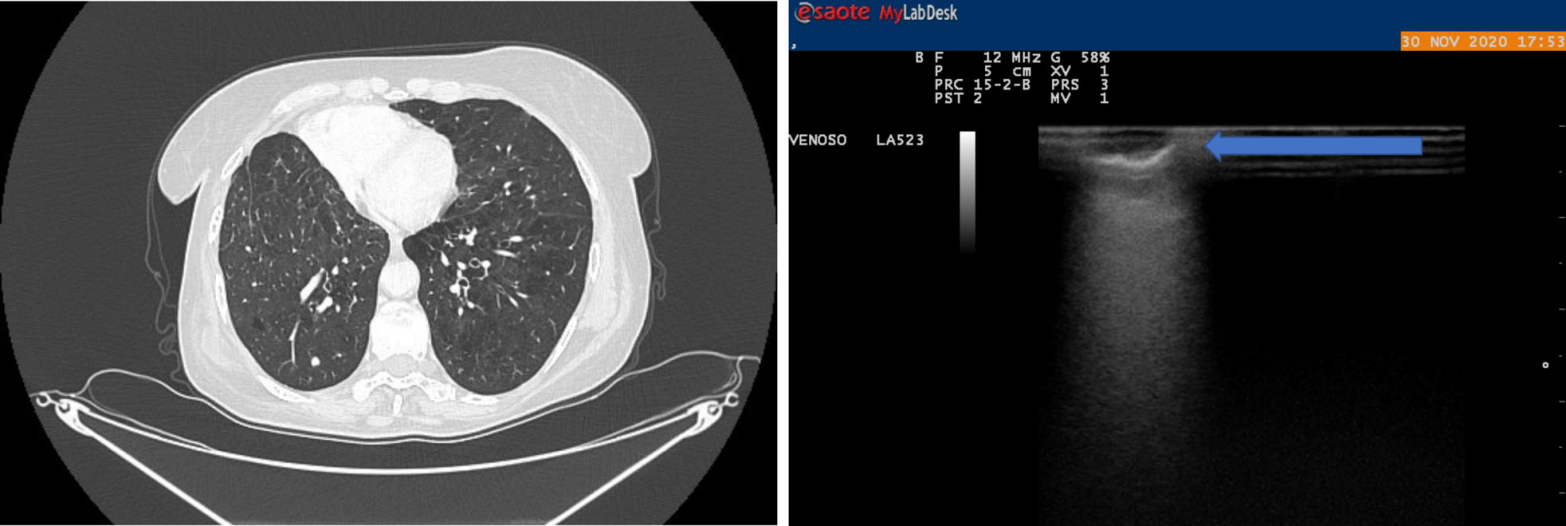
Irregular shaped lung nodule that was hypoecoic on ultrasound. Hyperecoic striae are present.
